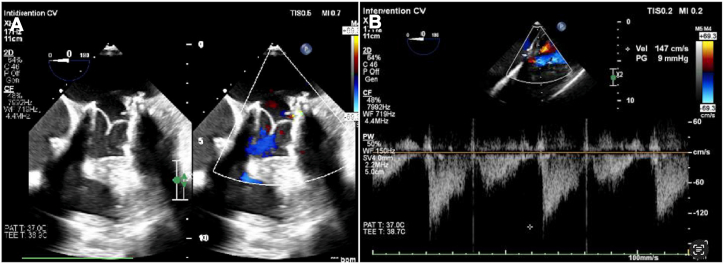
Final Result (A) Mild paravalvular leak on postdeployment transesophageal echocardiography. (B) Mean left ventricular outflow tract gradient of 9 mm Hg on postprocedural transthoracic echocardiography.
